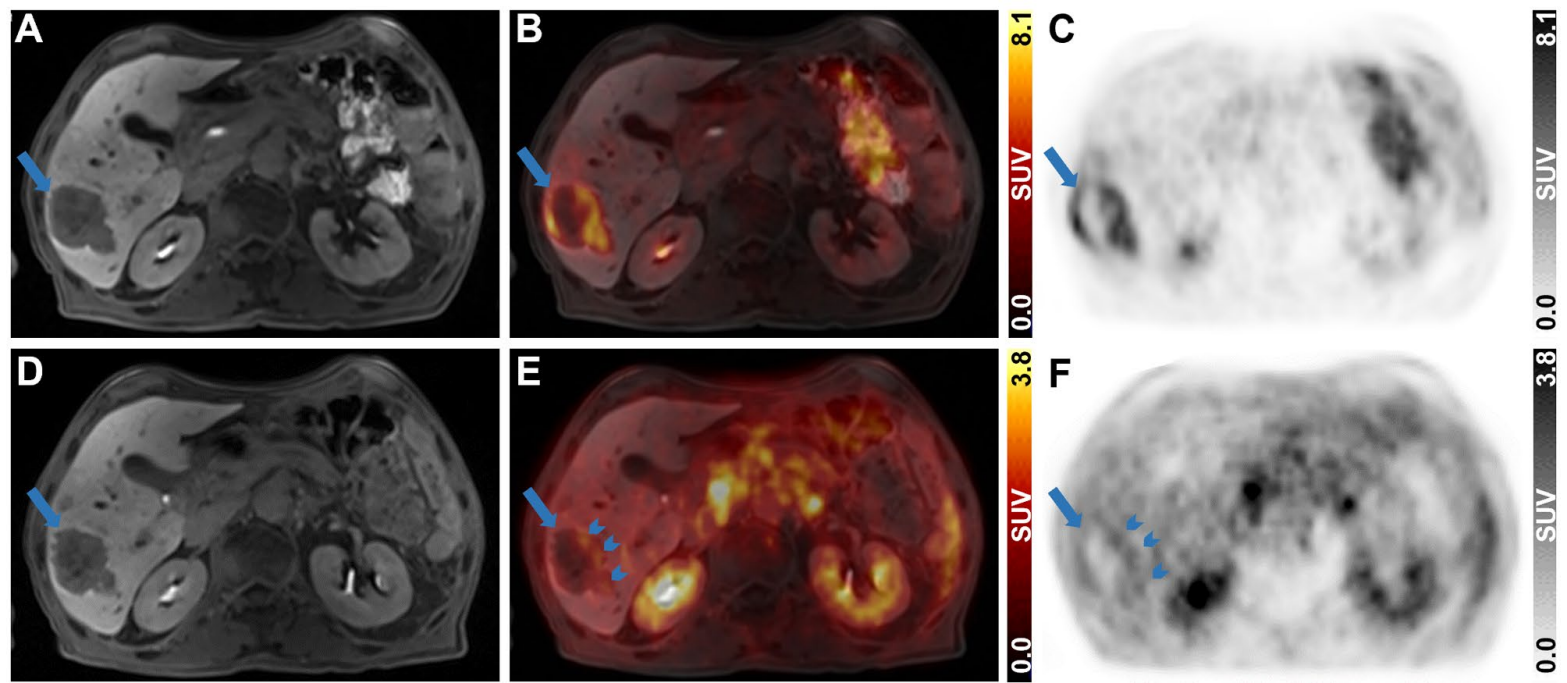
Diagnostic abdominal 2-[^18^F]FDG and [^68^Ga]Ga-Pentixafor PET/MR images of the liver metastasis in the CRC patient. (A, D) Contrast-enhanced T_1_-VIBE MR images, (C, F) PET images and (B, E) fused PET and MR images are presented in axial views for 2-[^18^F]FDG (105 min *p.i.*, 198 MBq, upper row) and [^68^Ga]Ga-Pentixafor (40 min *p. i.*, 158 MBq, lower row). Long blue arrows indicate the liver metastasis and short blue arrows indicate focal [^68^Ga]Ga-Pentixafor uptake
